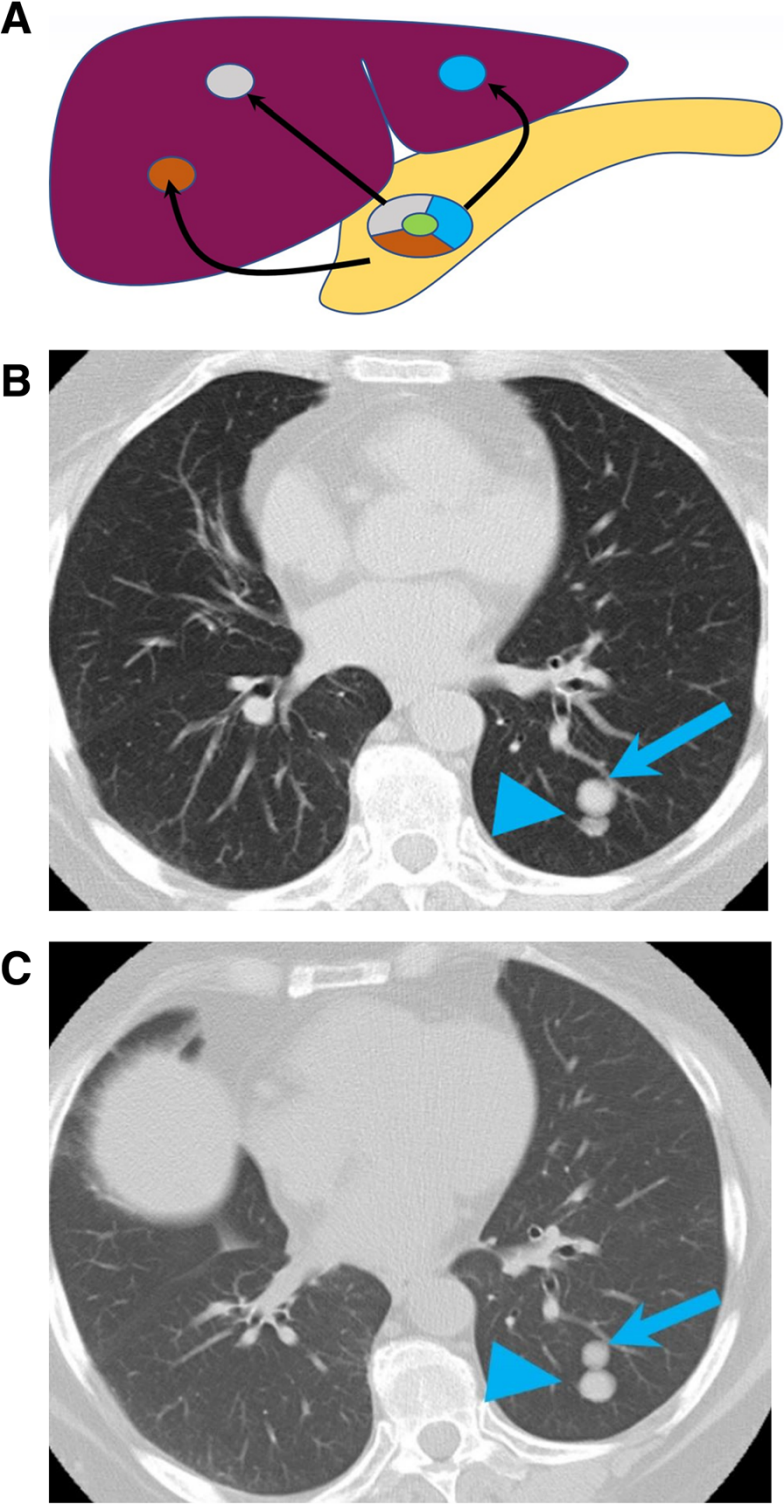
Intermetastatic heterogeneity. a Image showing the heterogeneity within different liver metastases, each one arising from a different subclone in a primary pancreatic tumor. b Baseline and (c) follow-up axial CT images of the chest of a 77-year-old woman with leiomyosarcoma on pazopanib shows increase in size of a nodule (blue arrowhead), and interval decrease in size of an adjacent nodule (blue arrow), representing different response to the vascular endothelial growth factor inhibitor pazopanib
